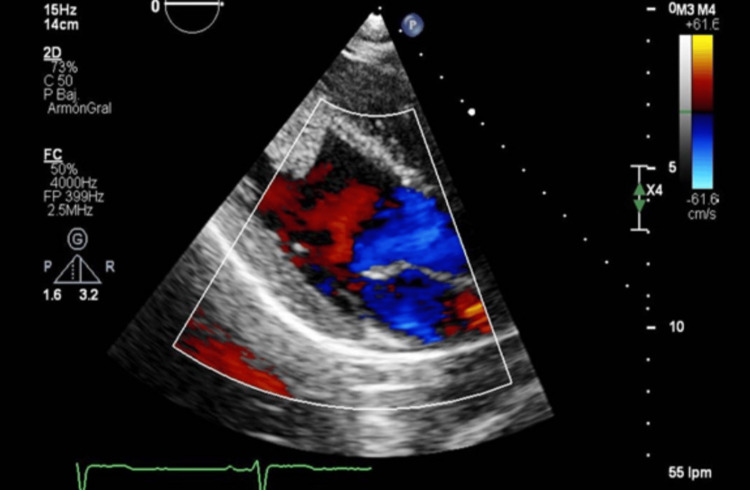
Color Doppler in long parasternal view showing preserved intracardiac flow without evidence of valvular dysfunction.
